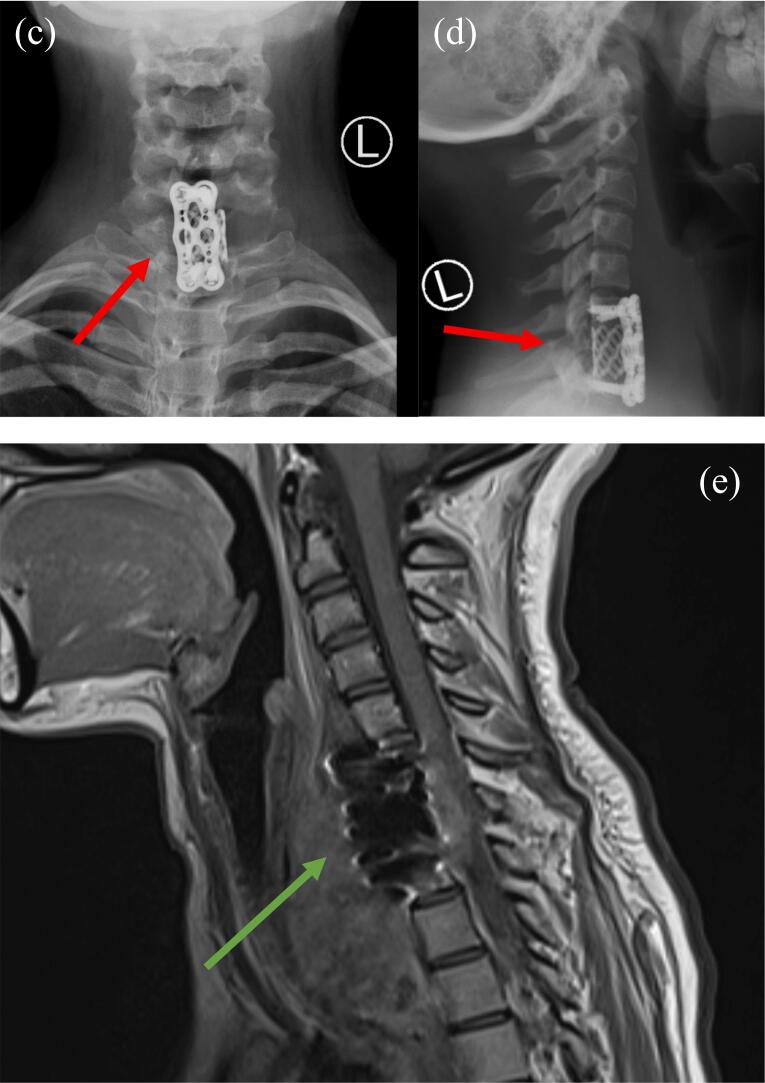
Postoperative radiographic assessment of the procedure and after the patient received radiotherapy. These images were taken after the treatment had been fully administered.
